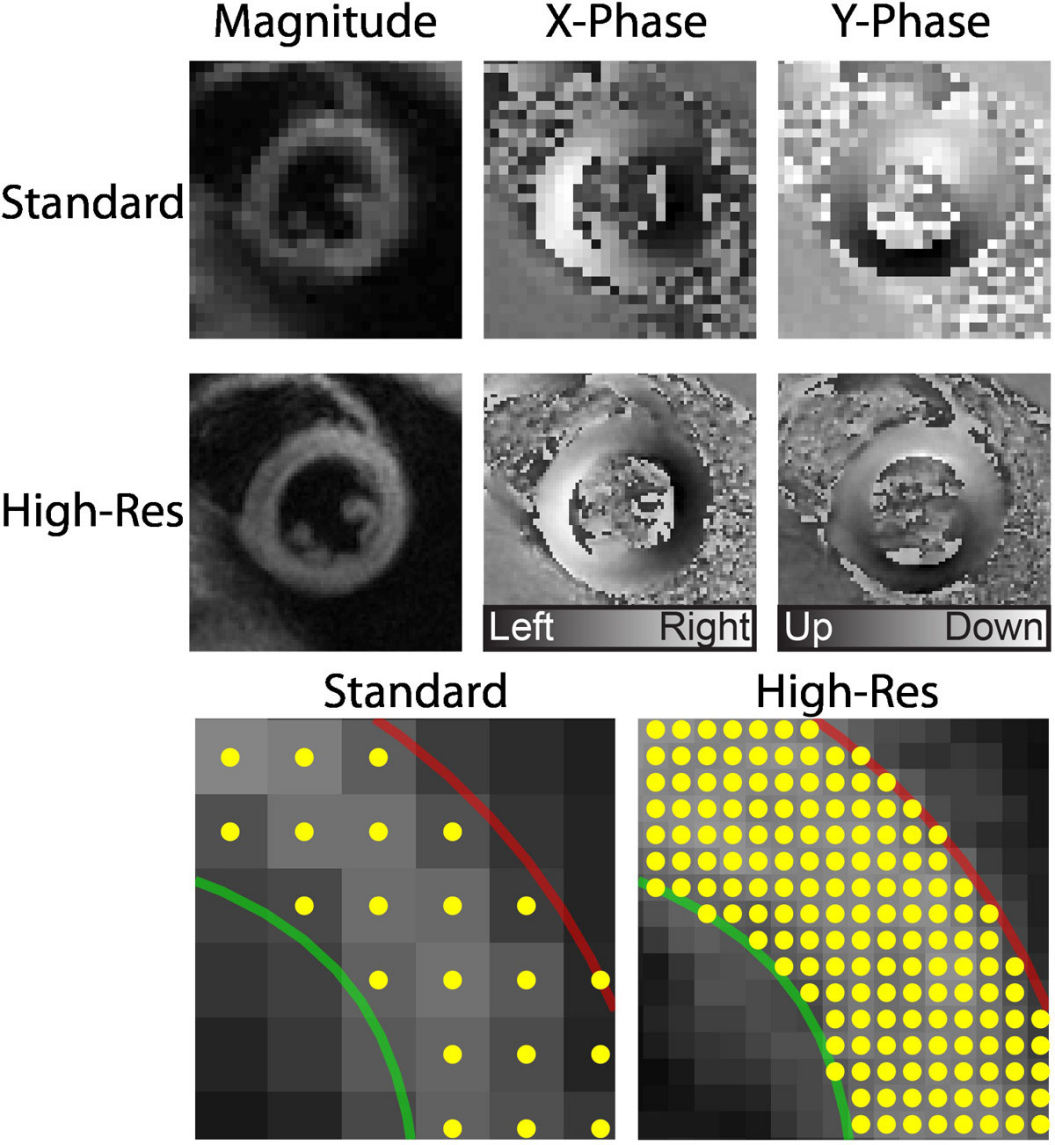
Comparison of standard and high resolution DENSE MRI.
